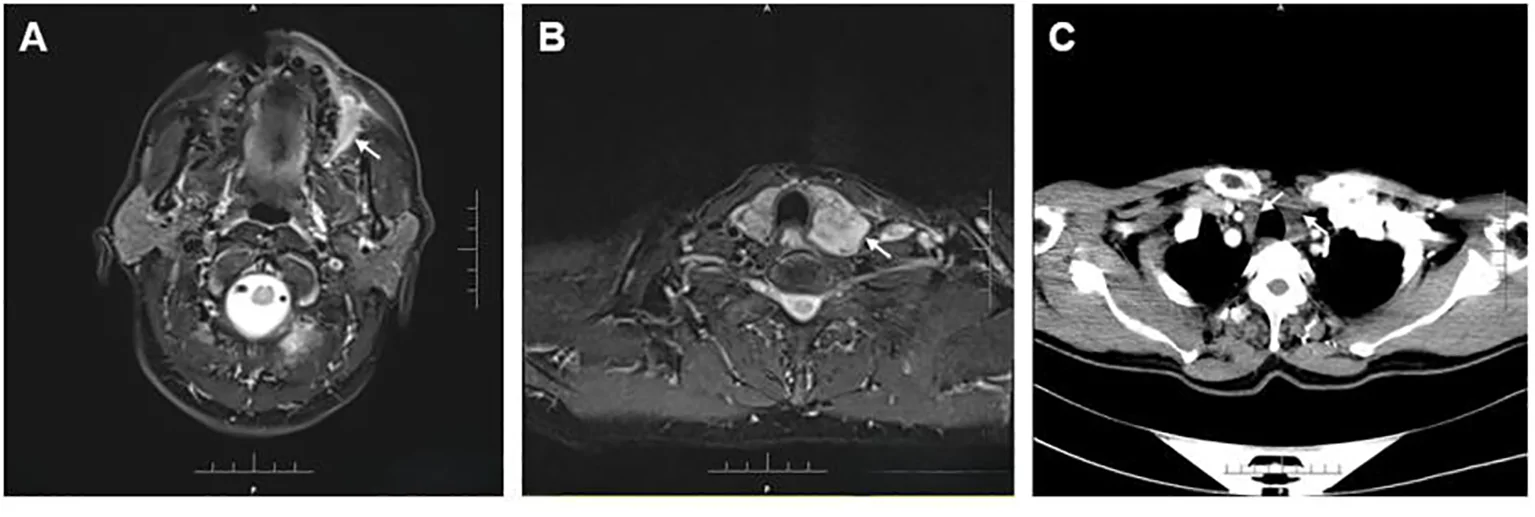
Neck MRI and CT images. (A) Primary lesion located in the left buccal region. (B) Enlarged thyroid gland with heterogeneous enhancement. (C) Multiple enlarged lymph nodes in level VI.
